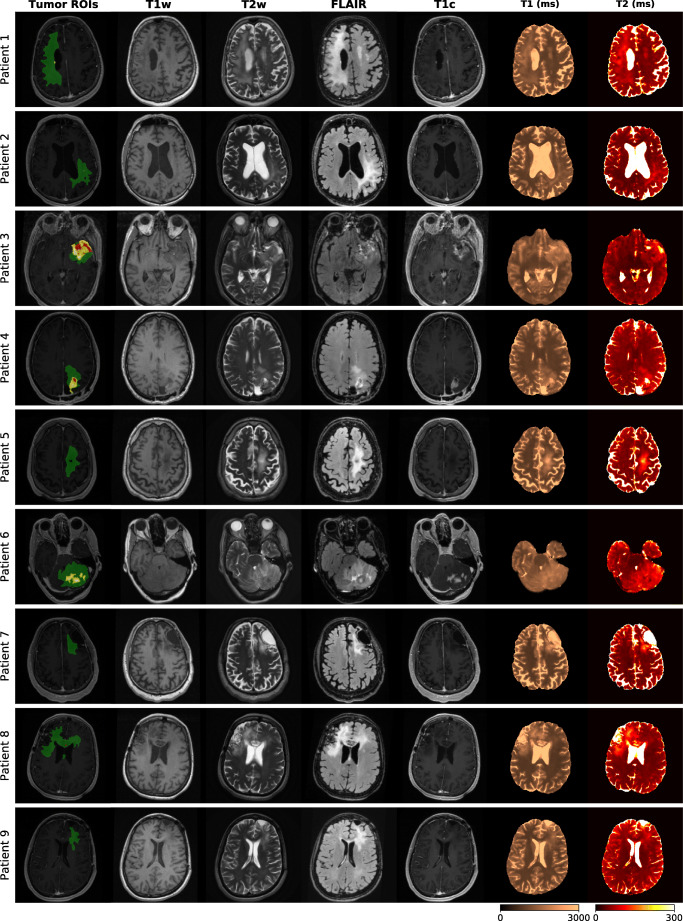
Qualitative comparison of tumor patient cases. Expert ROIs (green: peritumoral edema, red: necrotic core/non-enhancing tumor, yellow: enhancing tumor) are shown together with clinical T1-weighted FSPGR, T2-weighted, FLAIR, Gd-enhanced T1-weighted FSPGR images and quantitative T1 and T2 maps
